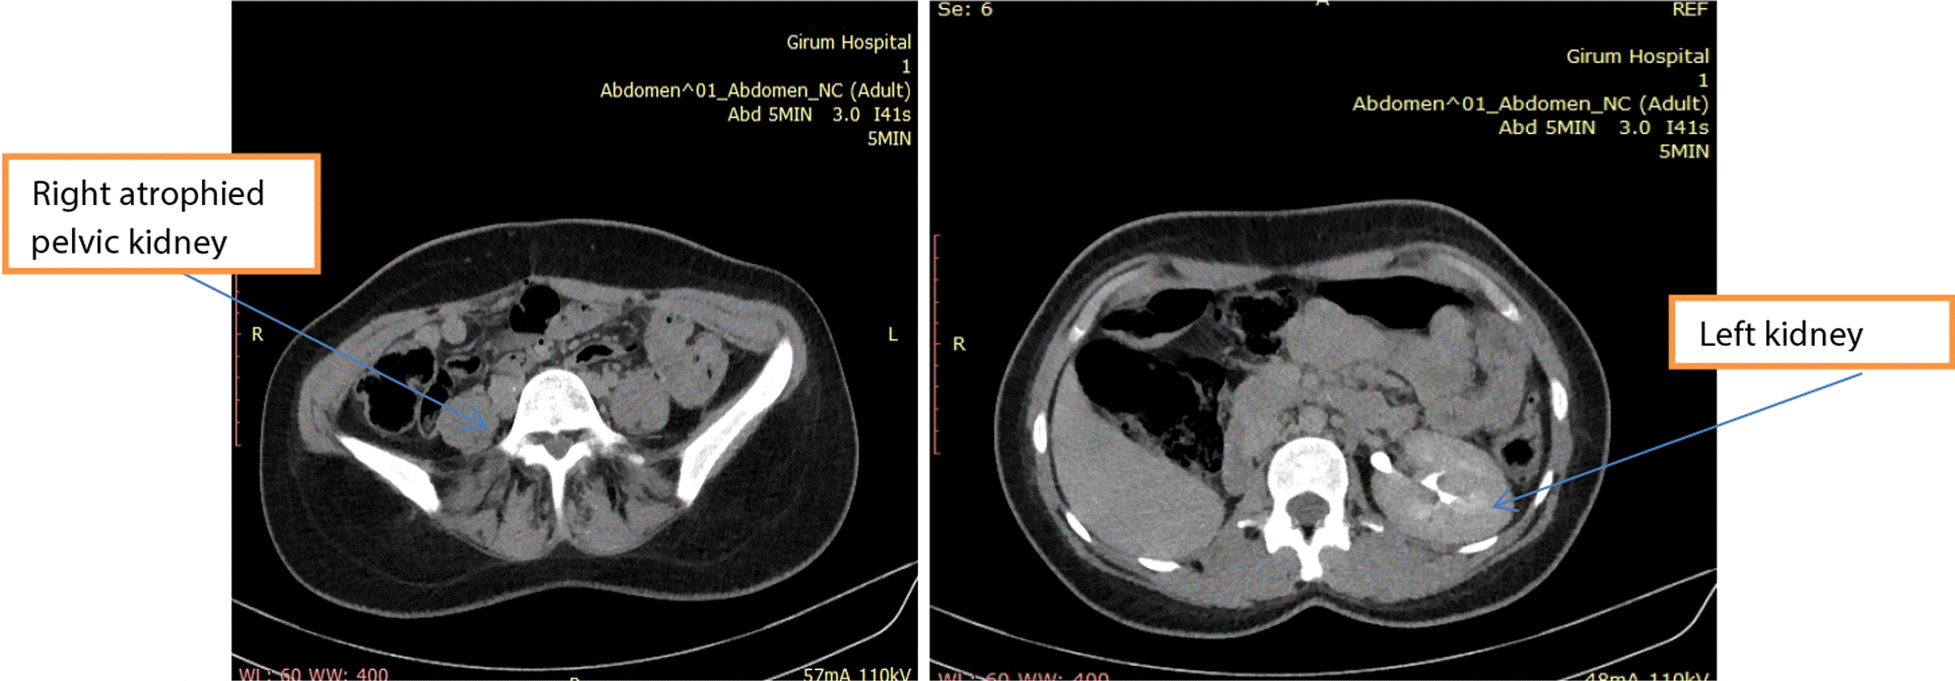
CT scan with contrast shows the right atrophied pelvic kidney and left normal kidney before surgery
